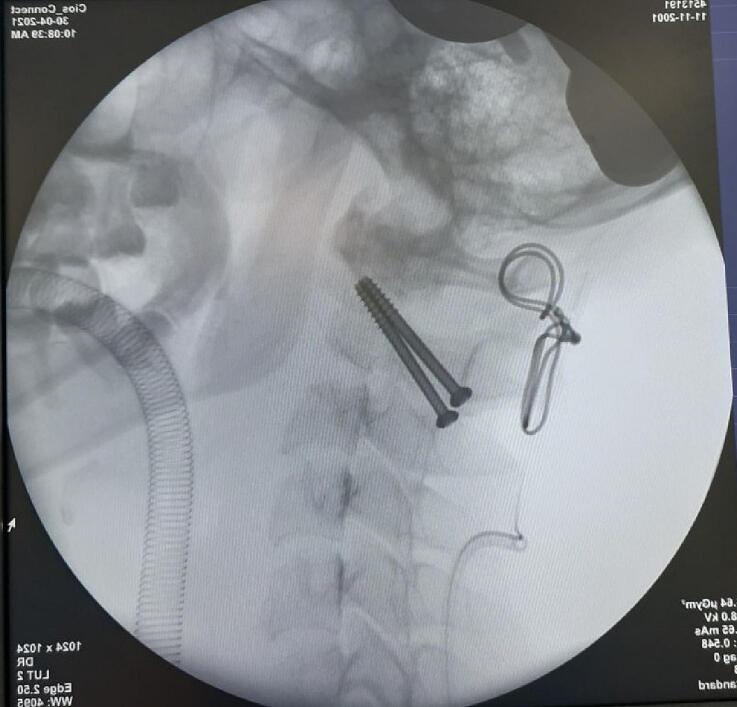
Postoperative X-ray cervical spine.
